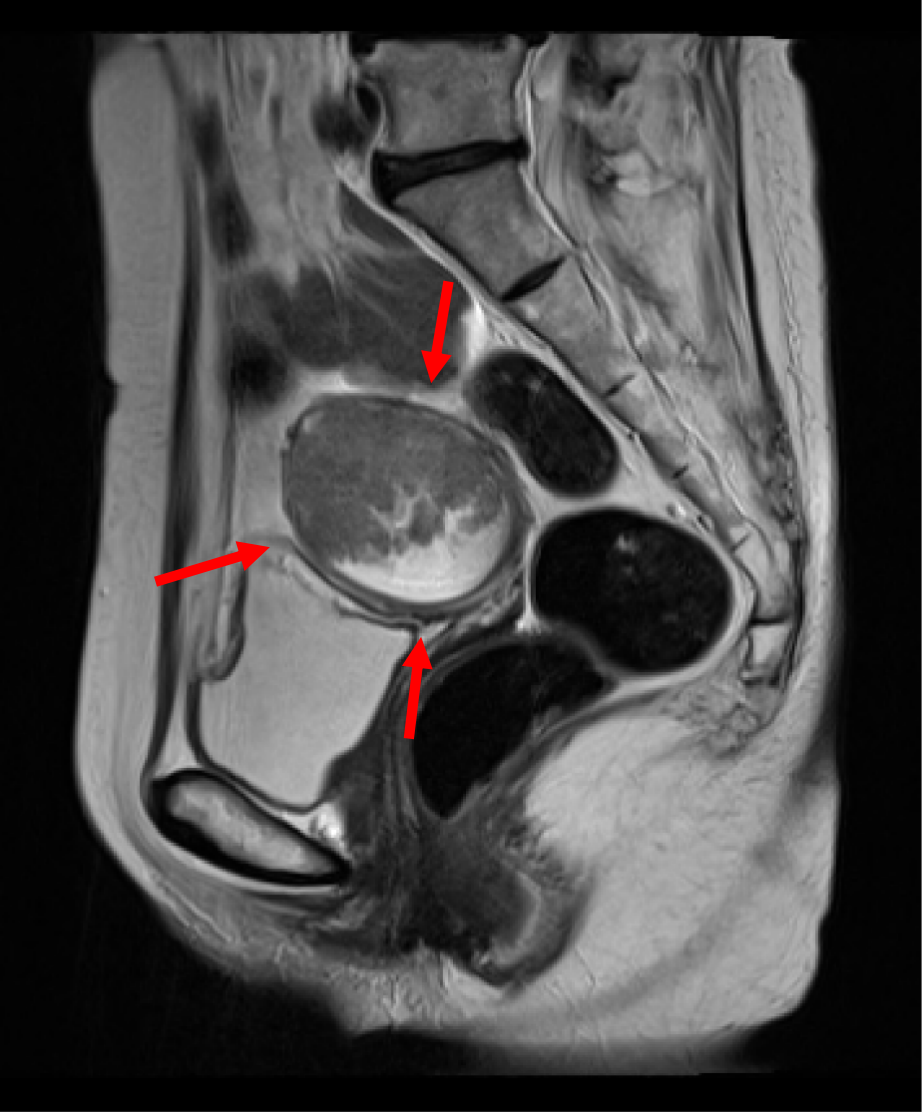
MRI shows invasion of the endometrial tumor into the outer half of the myometrium in the uterine corpus
